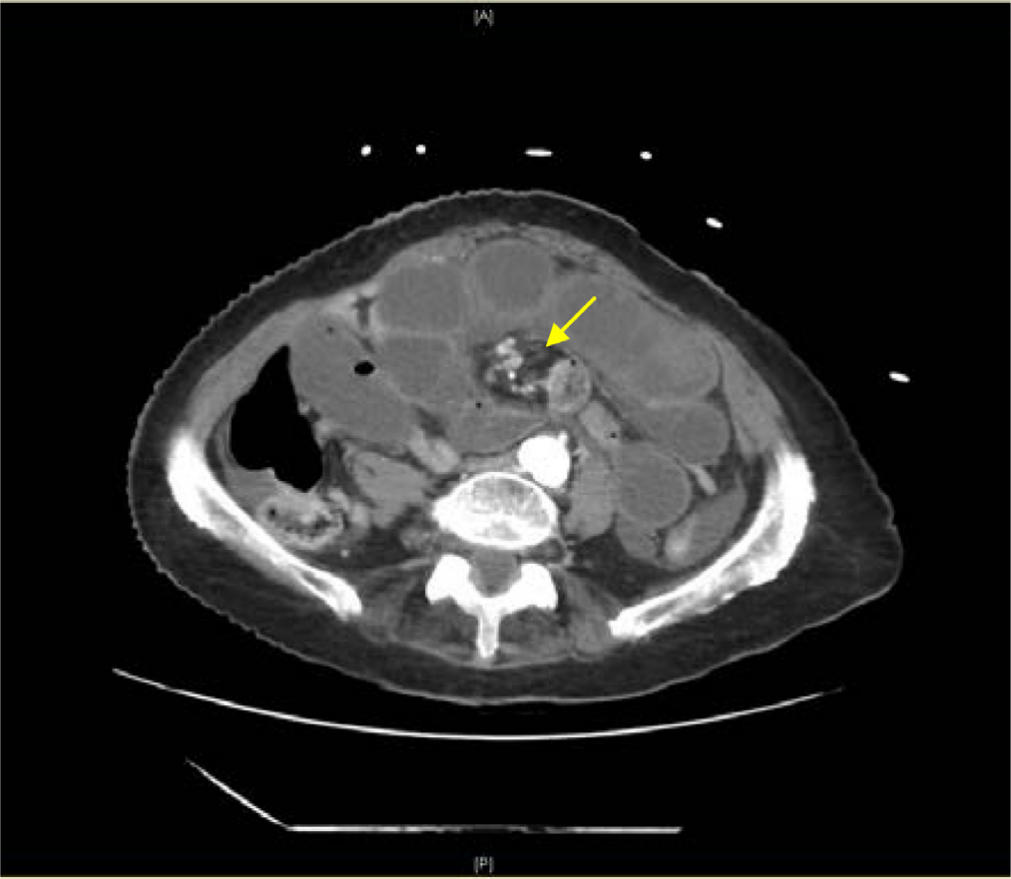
Computed tomography abdomen with intravenous contrast demonstrating dilated, fluid-filled loops of small bowel and a pattern of swirling mesenteric vessels called the whirlpool sign (arrow).
